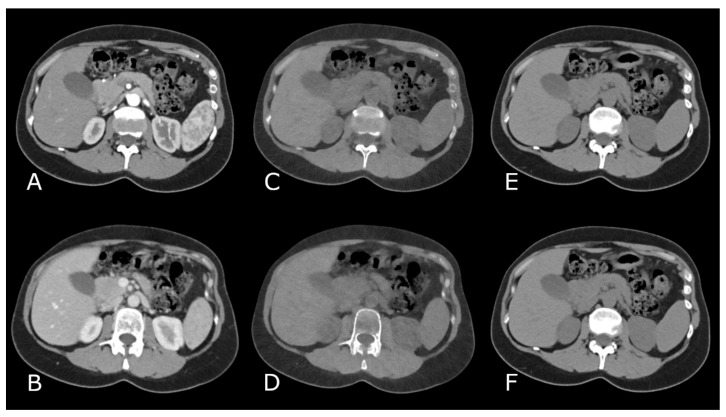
Images of a CT scan in arterial contrast phase (A) and in venous contrast phase (B) with corresponding virtual non-contrast images (C,D) and true non-contrast images from the same patient (E,F).
